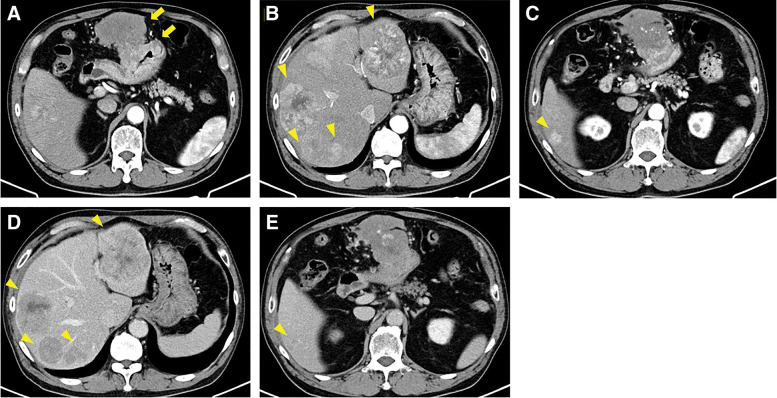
(A) Contrast-enhanced computed tomography showing thickening and enhancement of the gastric body wall with bulky lymph node swelling (arrows). (B–E) Five bilateral hepatic lesions, with the largest measuring 60 mm, exhibiting central necrosis and heterogeneous enhancement. All liver tumors demonstrated arterial hyperattenuation and washout on dynamic computed tomography (B and C, early phase; D and E, late phase; arrowheads).
